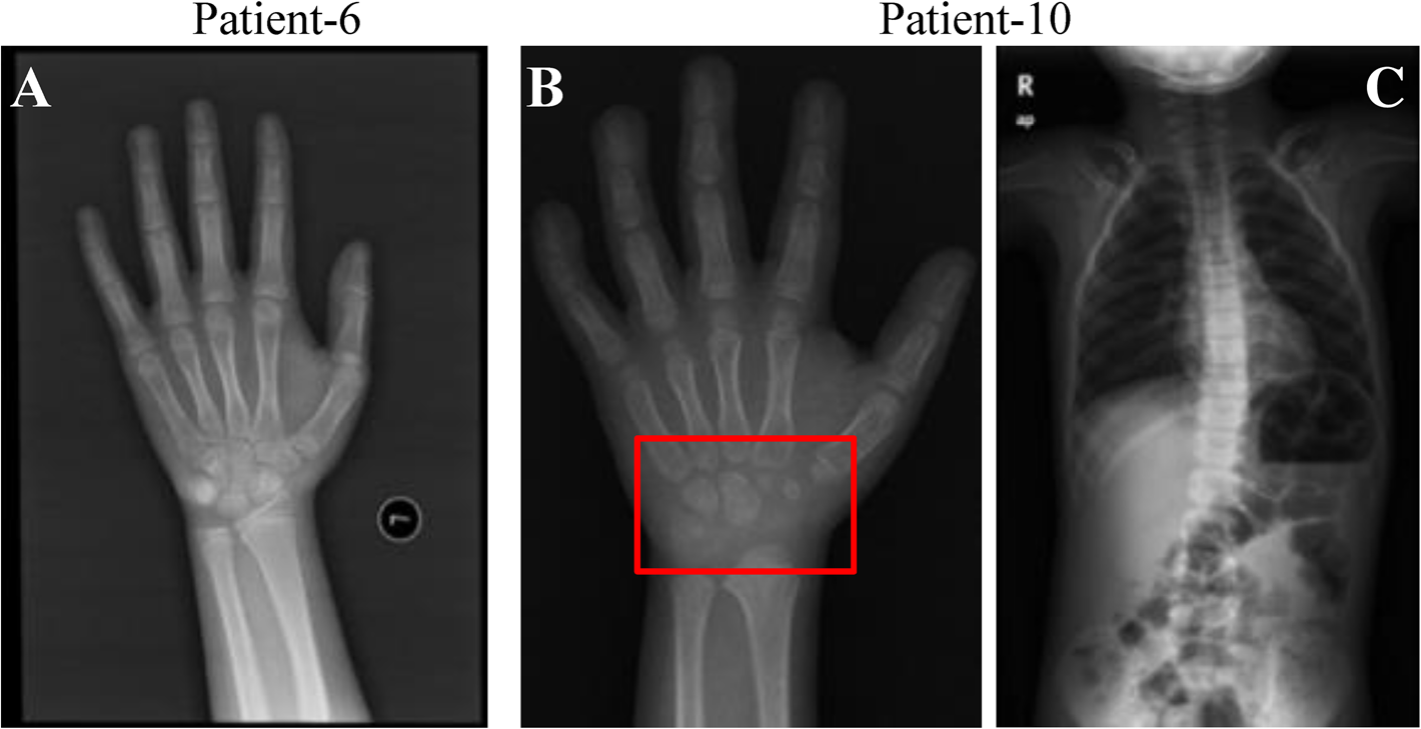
X-ray results of Patients 6 and 10. a The bone age of Patient 6 was advanced for 2.5 years. Patient 10 had a bone age of 4.5–5 years old and severe carpal epiphyseal growth retardation (b) and scoliosis (c)
